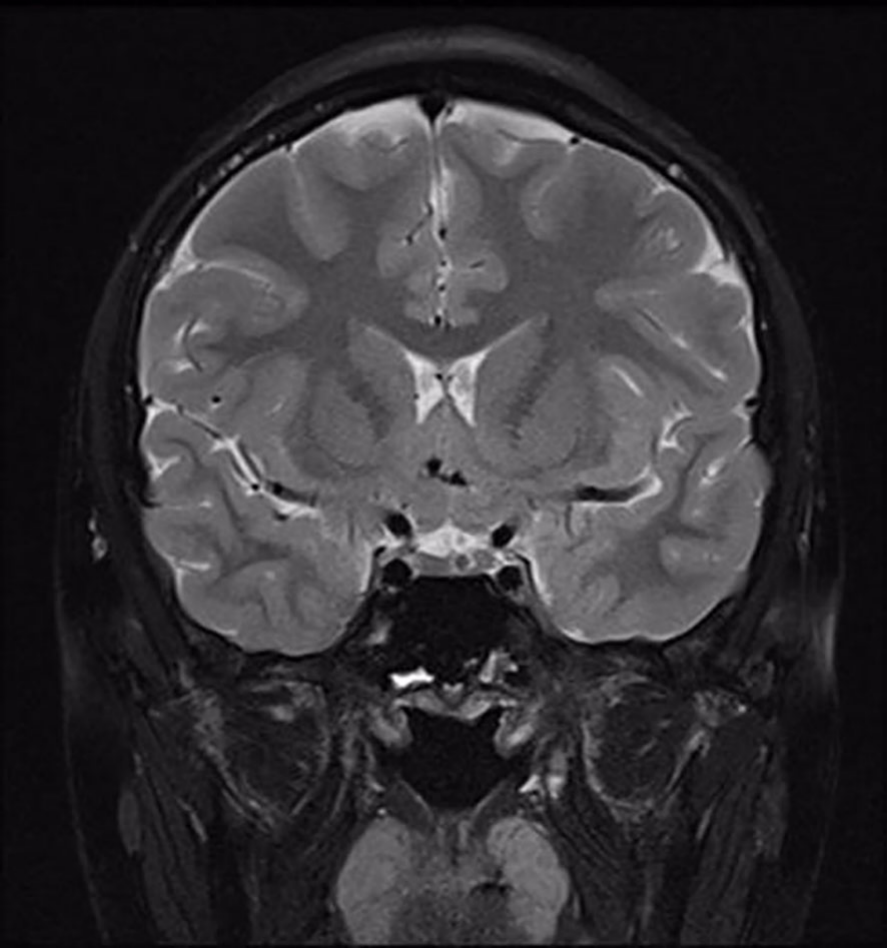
MRI image of the pituitary gland. A nodule in the lower left pituitary is observed.
